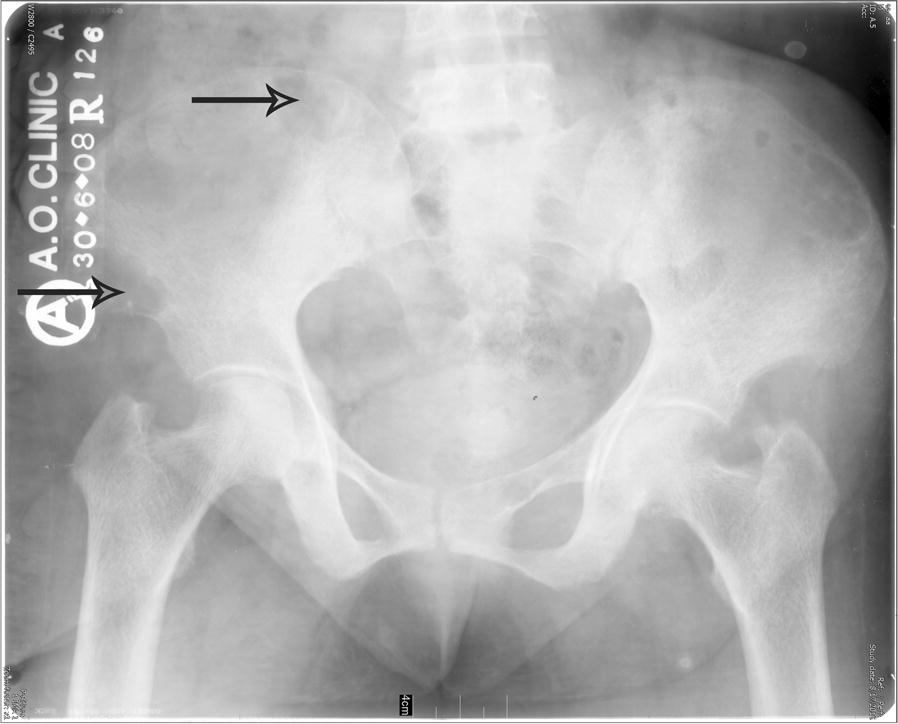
X-ray pelvis (punched out lytic lesions in right iliac bone)
